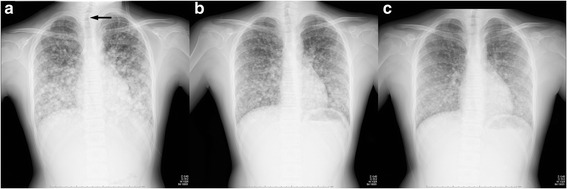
Chest radiographs. a Chest radiograph showing innumerable lung nodules in a miliary pattern. The trachea is deviated to the right (*arrow*). b In 2 months after receiving sorafenib and before surgery showing the relief of deviated trachea. c In 2 month after surgery and resume sorafenib showing improvement in the lung nodules
